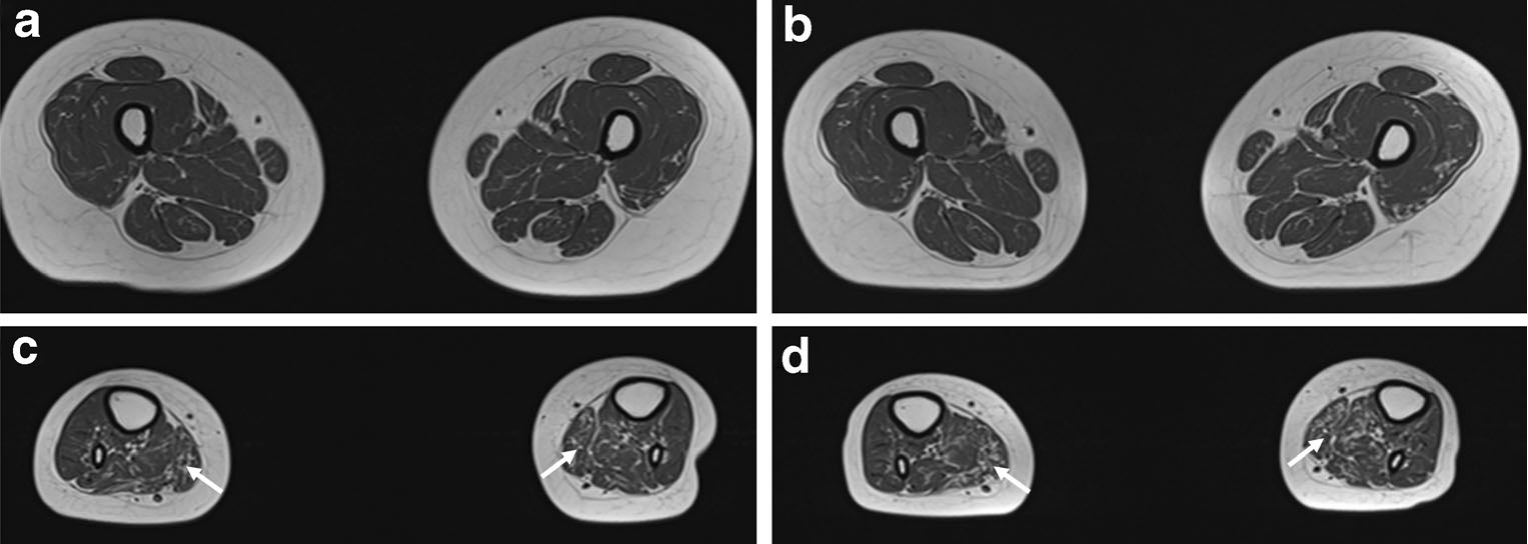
Lower limb MRIs in patient II-1. MRIs of the thigh (a, b) and lower leg (c, d) at 20 (a, c) and 23 (b, d) years of age. Follow-up studies revealed progression of atrophic changes. a, b At the thigh level, T1-weighted images demonstrated relatively intact muscle without signal changes or atrophies. c, d In the lower leg, MRIs revealed moderate involvement of superficial posterior compartment muscles, including the soleus and gastrocnemius muscles (*arrows*); however, anterior and lateral compartment muscles were spared
